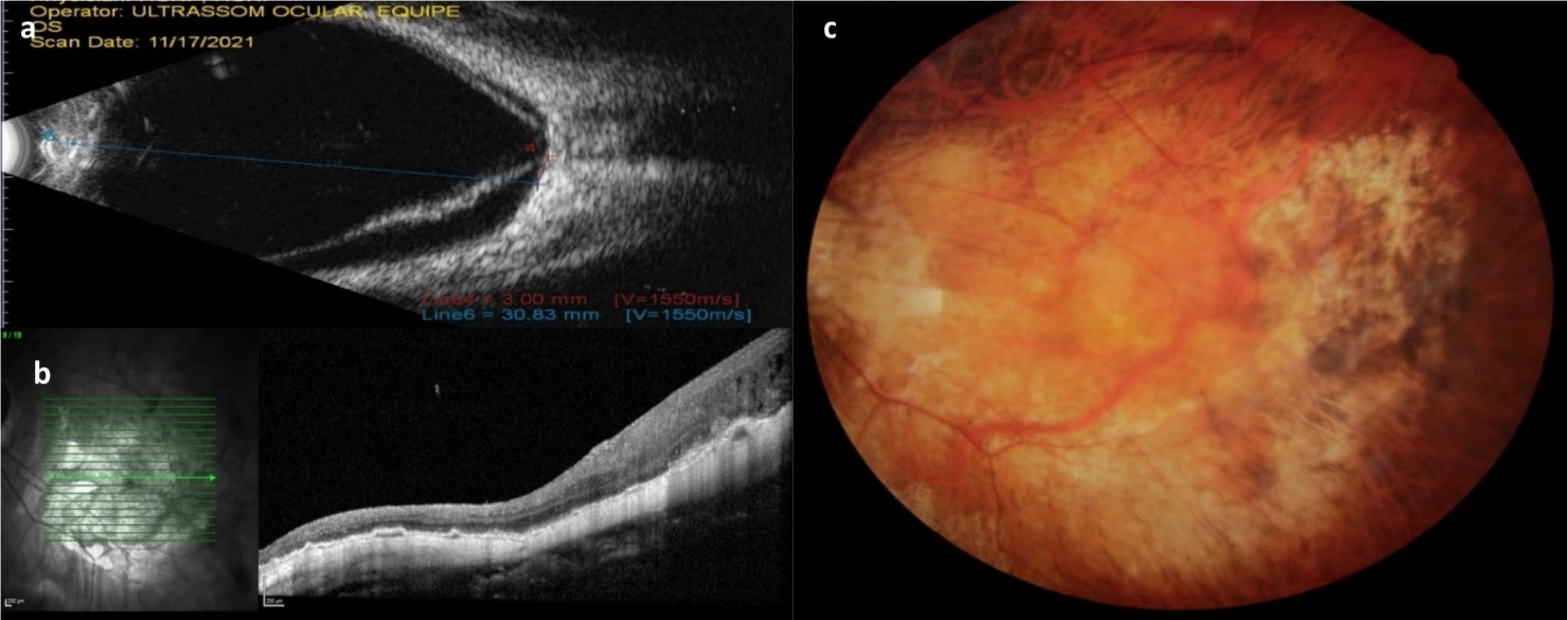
a: Ultrasound of the left eye shows retinal detachment; b: Postoperative OCT reveals attached retina; c: Postoperative color fundus photography of the left eye demonstrates a reattached retina
